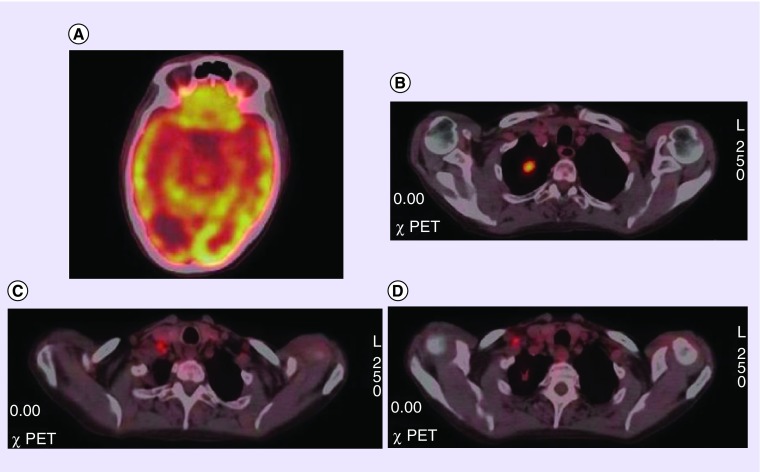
F-18 F-18 fluorodeoxyglucose PET/CT image of a patient with hipometabolic brain lesion. (A) F-18 fluorodeoxyglucose PET/CT axial images of brain region showing hypometabolic metastatic brain lesion. (B) Hypermetabolic lung lesion in the apex of right lung. (C & D) Lymph node metastasis in neck (level 6) and right supraclavicular region.
